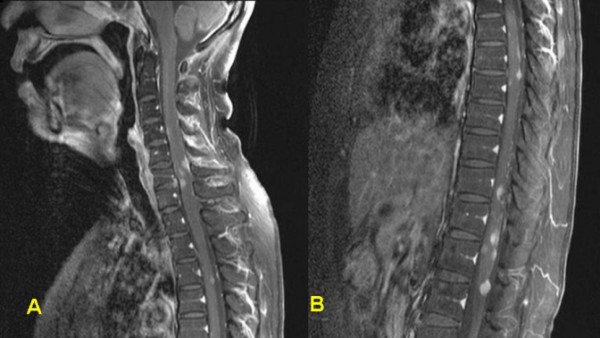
Spinal sagittal MR images (A: cervical, B: thoracic) revealing multiple nodular enhancing masses on the cervico-thoracic area.
